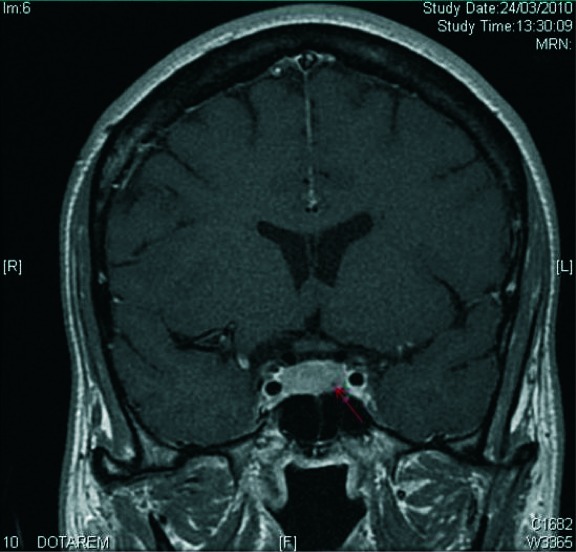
Coronal T1-weighted postcontrast MRI of pituitary showing enlarged pituitary with a small microadenoma or cyst (indicated by arrow).
